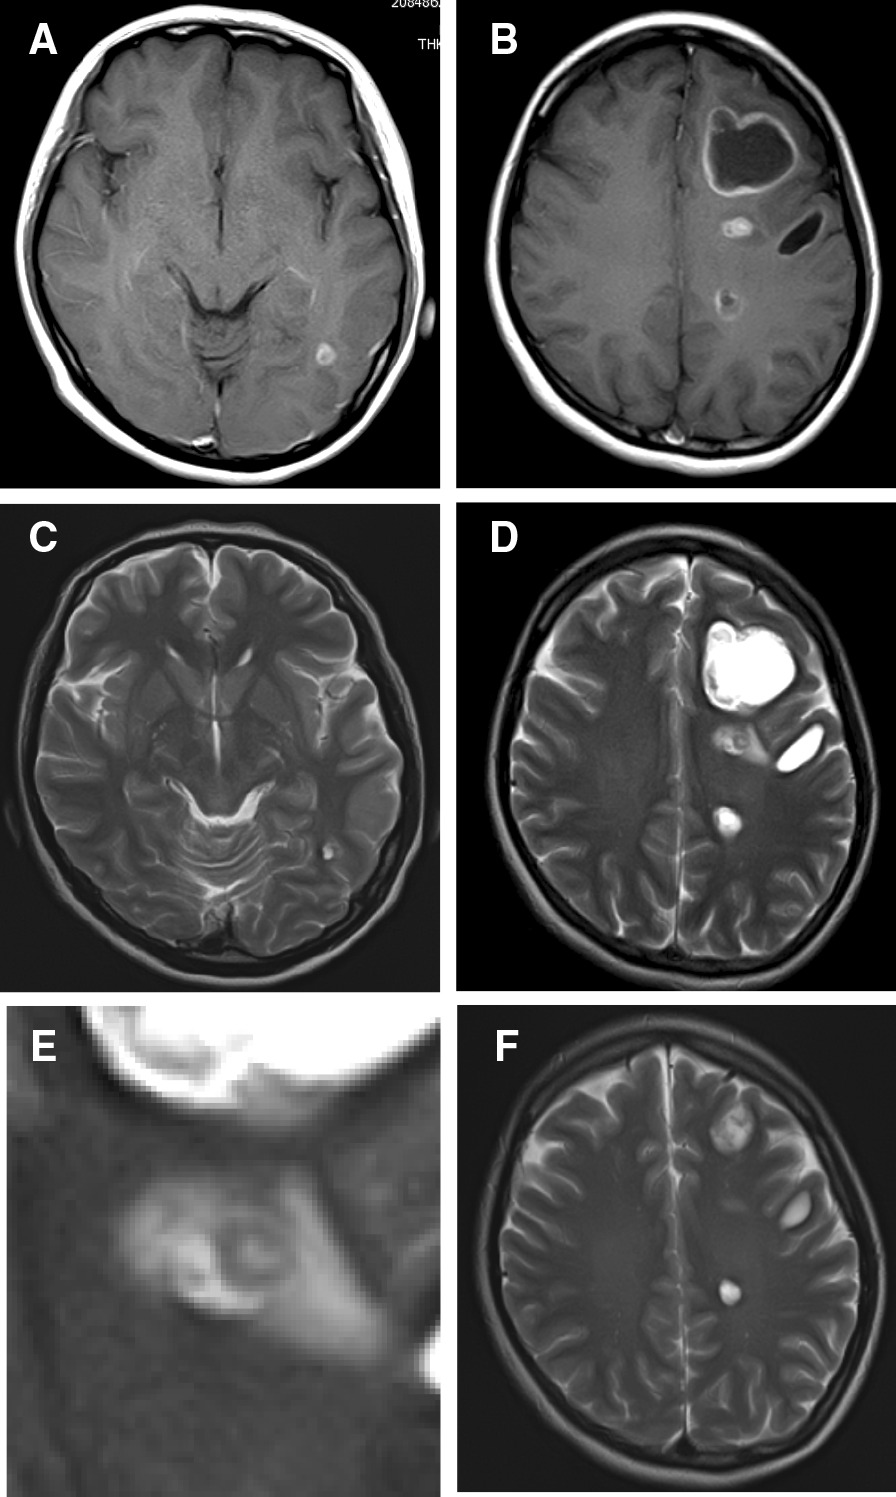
Brain MRI findings. A, B Gadolinium-enhanced brain MRI revealed strong ring enhancement of cystic lesions. C, D T2-weighted image showing some perilesional focal edema. E T2-weighted image revealing a scolex inside the cyst located outside the left lateral ventricle. F A brain MRI scan acquired 7 months after the start of ABZ treatment demonstrated multiple small well-defined cystic and nodular structures of different sizes without perilesional edema or calcification
